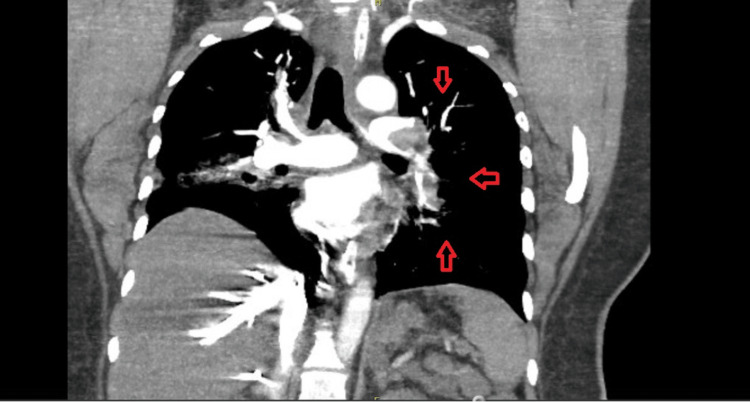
CT-pulmonary angiography: arterial phase revealing a thrombus causing significant luminal stenosis of the left main pulmonary artery
